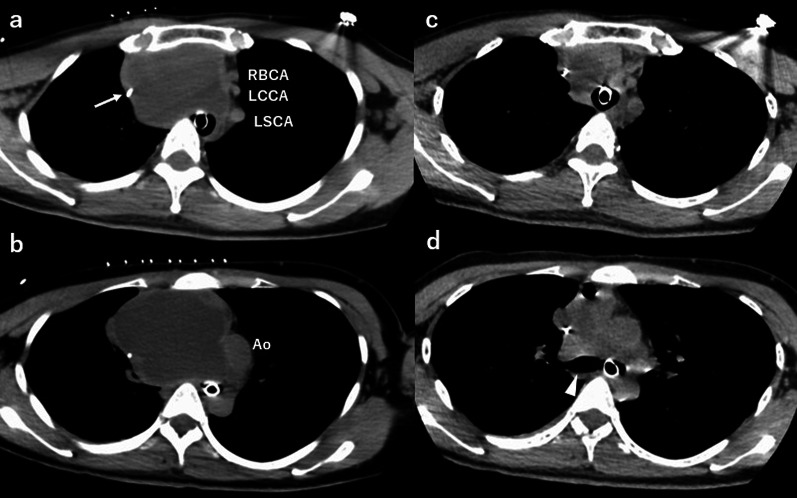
Comparison of CT images before and after drainage. A double-lumen tube was intubated into the left main bronchus. Central venous catheter was inserted from the right internal jugular vein (white arrow). The images a and b were taken before drainage. The images c and d were taken after drainage. The images a and c are at the trachea level and images b and d are at the level of carina of the trachea. After drainage, the right main bronchus was patent (white arrowhead). *CT* computed tomography, *RBCA* right brachiocephalic artery, *LCCA* left common carotid artery, *LSCA* left subclavian artery, *Ao* aorta
